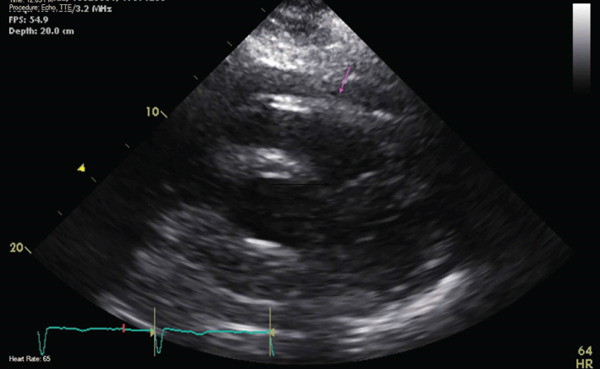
Echocardiogram showing moderate pericardial effusion and hyperdynamic left ventricle as pointed out by red arrows.
